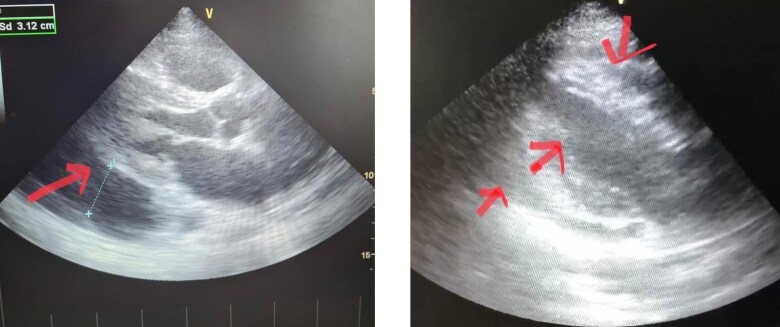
Transthoracic echocardiogram before pericardiocentesis demonstrating a large pericardial effusion.
